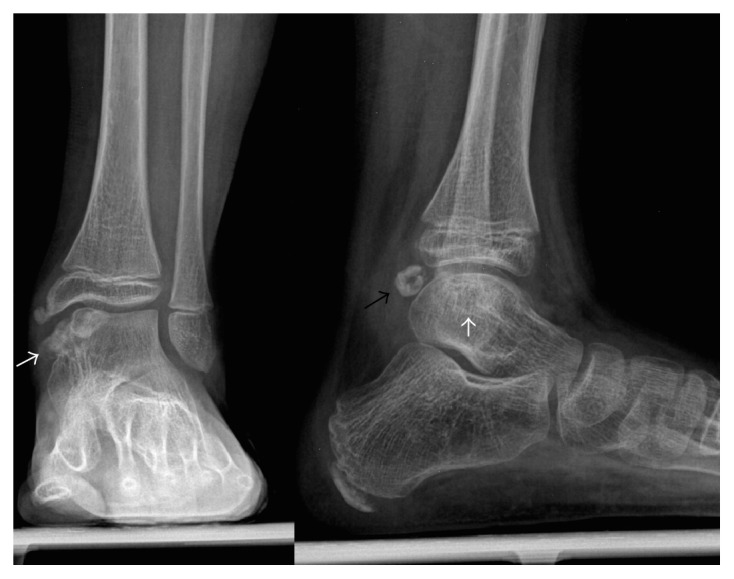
X-rays show medial talus and tibia bony formations (white arrows) and posterior cauliflower fashioned loose body (black arrow).
